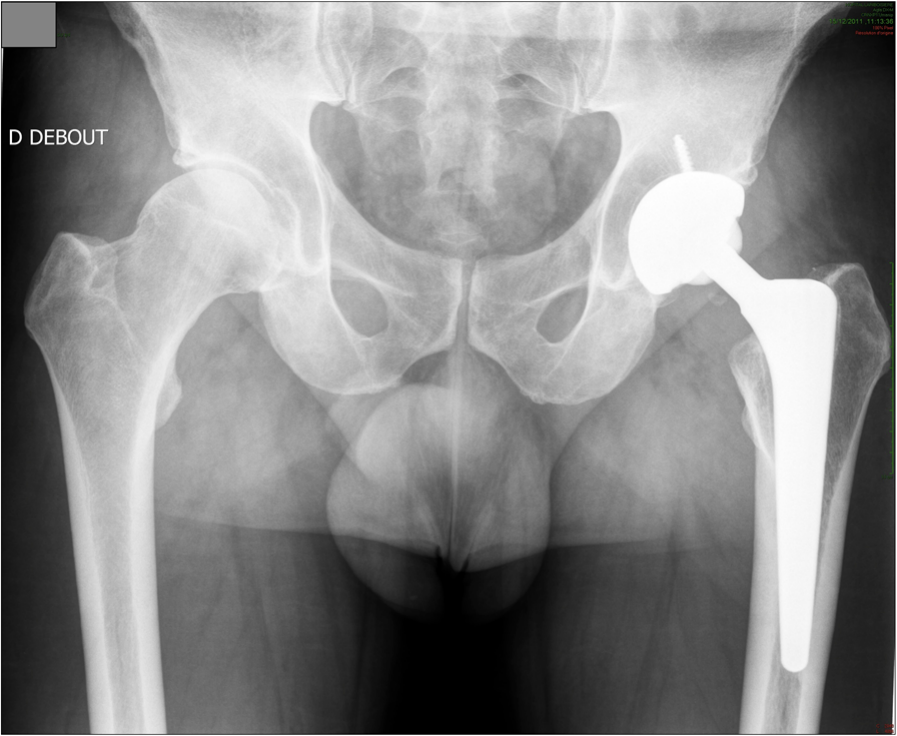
Pelvic post operative X-ray
